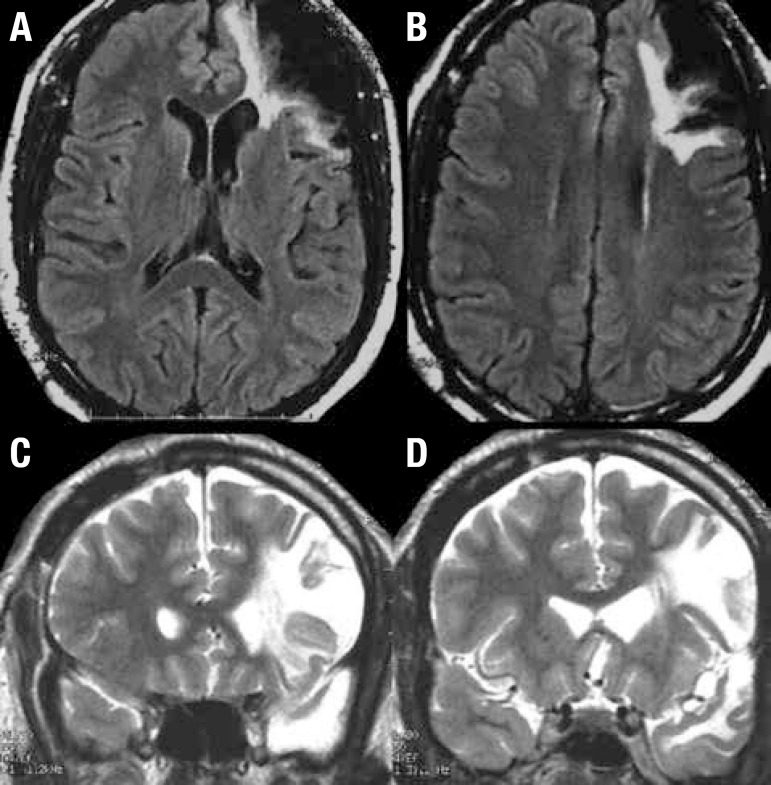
Brain MRI. [A, B] Axial FLAIR. [C, D] coronal T2WI. Reveals a large area
